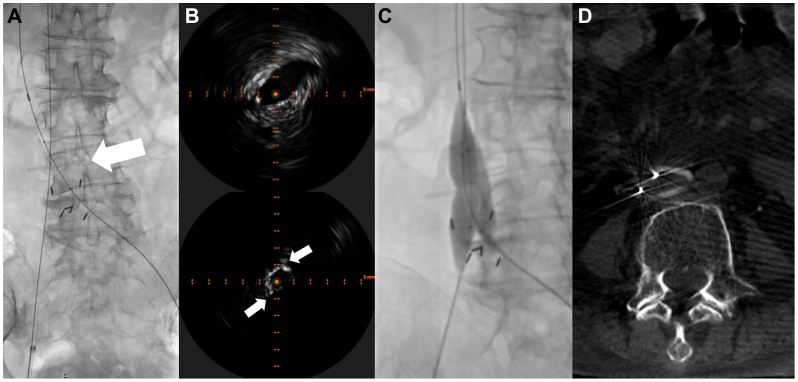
The patient was taken for endovascular venography and intervention. (A) In a prone position, bilateral popliteal venous access was obtained and the left lower extremity venous thrombosis as well as the inferior vena cava (IVC) clip was crossed. The Moretz clip is faintly visible (*white arrow*). (B) IVUS images demonstrate normal-appearing IVC (top) and echogenic linear signal in keeping with the Moretz clip (bottom; *white arrows*). (C) Balloon venoplasty is performed with two, 14-mm angioplasty balloons in a kissing fashion. (D) A cone beam computed tomography (CT) scan was performed, demonstrating deformation and expansion of the clip into more of an oval configuration.
